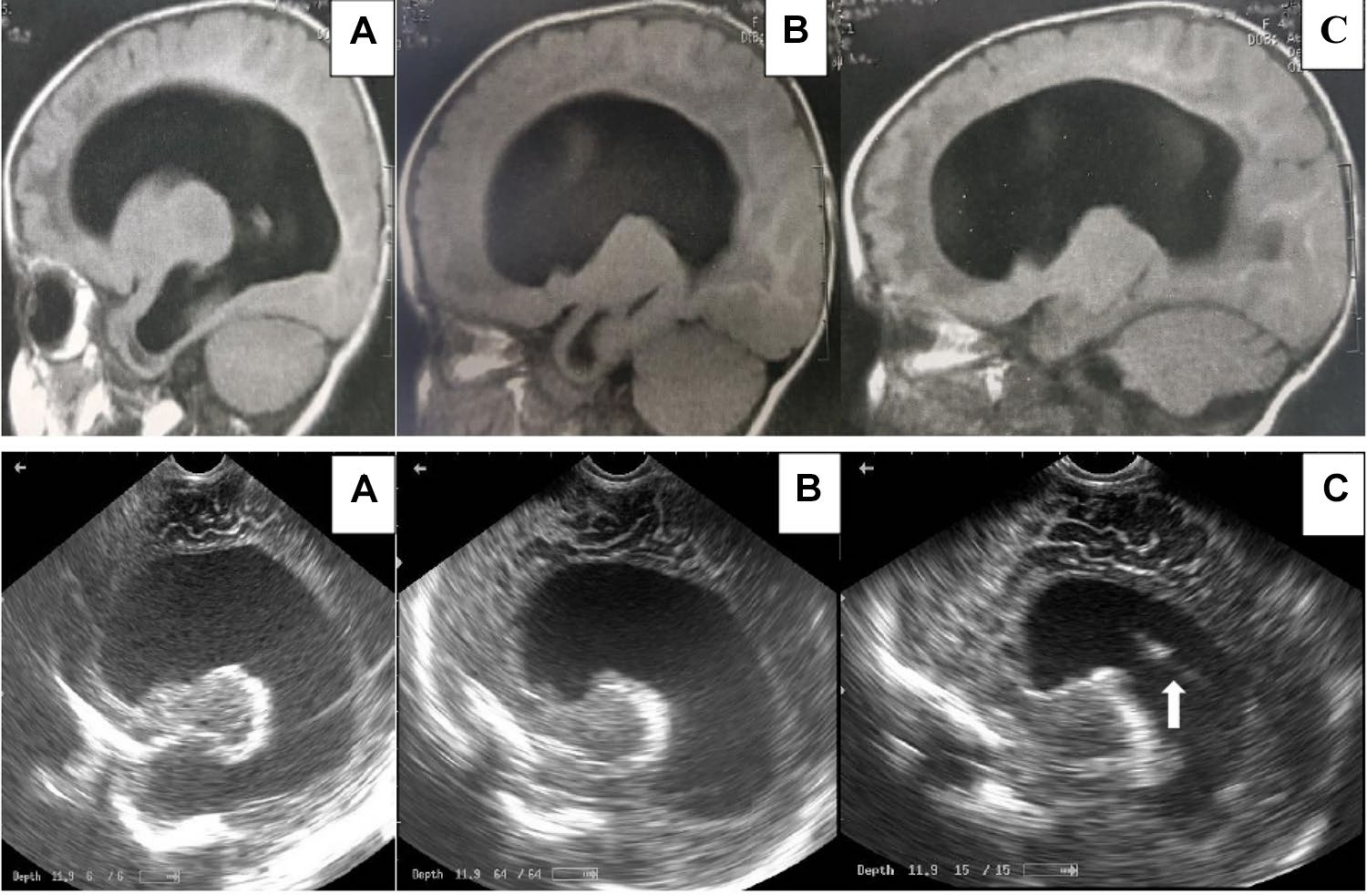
The ability of ultrasound to capture images at different sagittal levels equivalent to an MRI of the same patient. Note the catheter ( arrowed) in the last ultrasound image C
